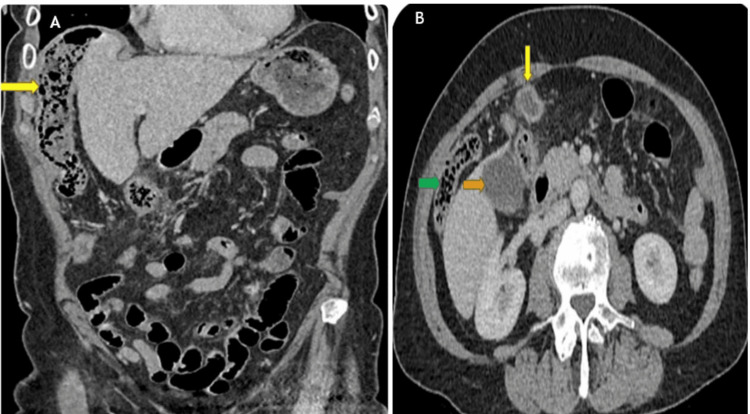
CT of the abdomen and pelvis A: Coronal view shows Chilaiditi syndrome with the colon interposed between the diaphragm and liver (yellow arrow); B: Sagittal view shows the right colon trapped between the diaphragm and liver (green arrow), gallbladder (orange arrow), and appendix (yellow arrow)
